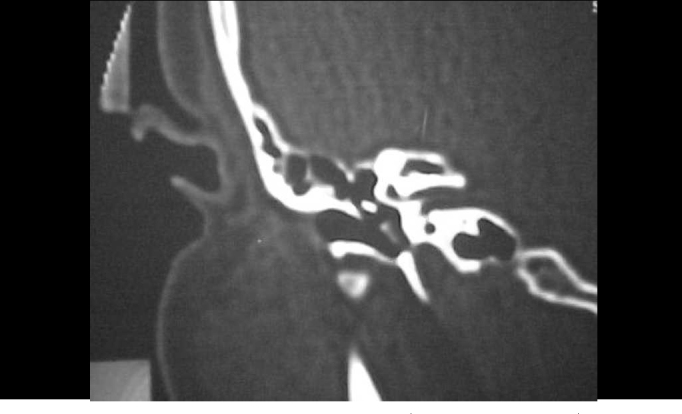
CT scan of temporal bone (coronal section) showing circumscribed velamentum of the right promontory and hypotympanum.
